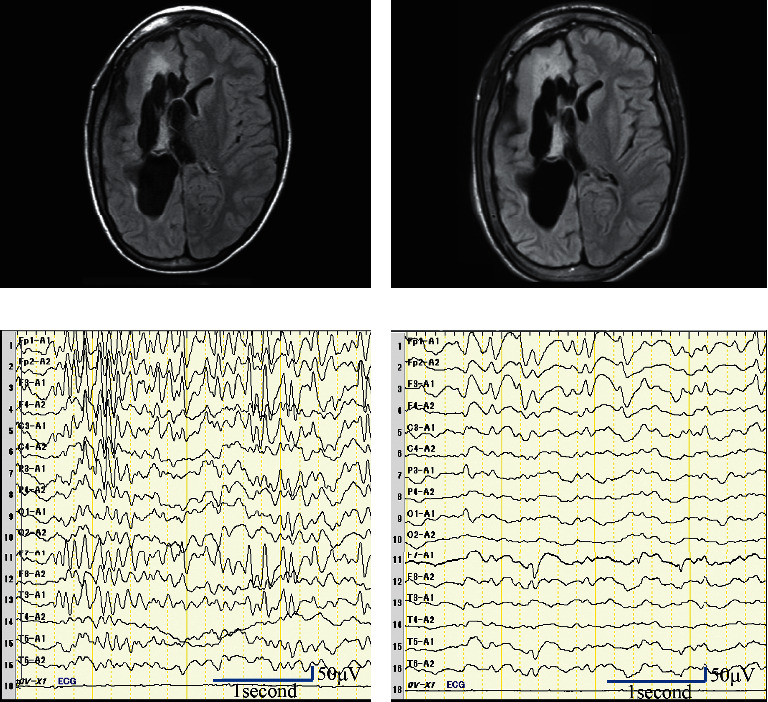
(a) MR image using fluid-attenuated inversion recovery (FLAIR) at 9 years 4 months. (b) Repeat FLAIR image obtained at 15 years 11 months (on day 7 after infection onset). (c) Electroencephalogram obtained during sleep at 15 years 1 month. (d) Electroencephalogram obtained during the period of impaired consciousness at 15 years 11 months. MR, magnetic resonance.
